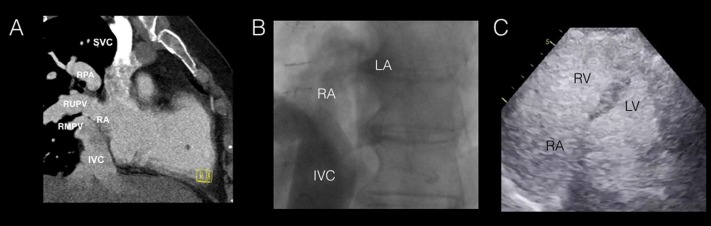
Multimodality Cardiac Imaging with Computed Tomography, Catheterization, and Echocardiography (A) Contrast-enhanced paracoronal computed tomographic image confirming the anomalous drainage of the right superior and right middle pulmonary veins into the right atrium; (B) IVC imaging during cardiac catheterization demonstrated filling of the right and left atria with the suggestion of an inferior defect in the interatrial septum; (C) An apical four chamber view on transthoracic echocardiography demonstrating significant opacification of both right and left atria following the administration of agitated saline contrast in the pigtail catheter within the IVC. IVC: inferior vena cava; LA: left atrium; LV: left ventricle; RA: right atrium; RMPV: right middle pulmonary vein; RPA: right pulmonary artery; RUPV: right upper pulmonary vein; RV: right ventricle; SVC: superior vena cava
